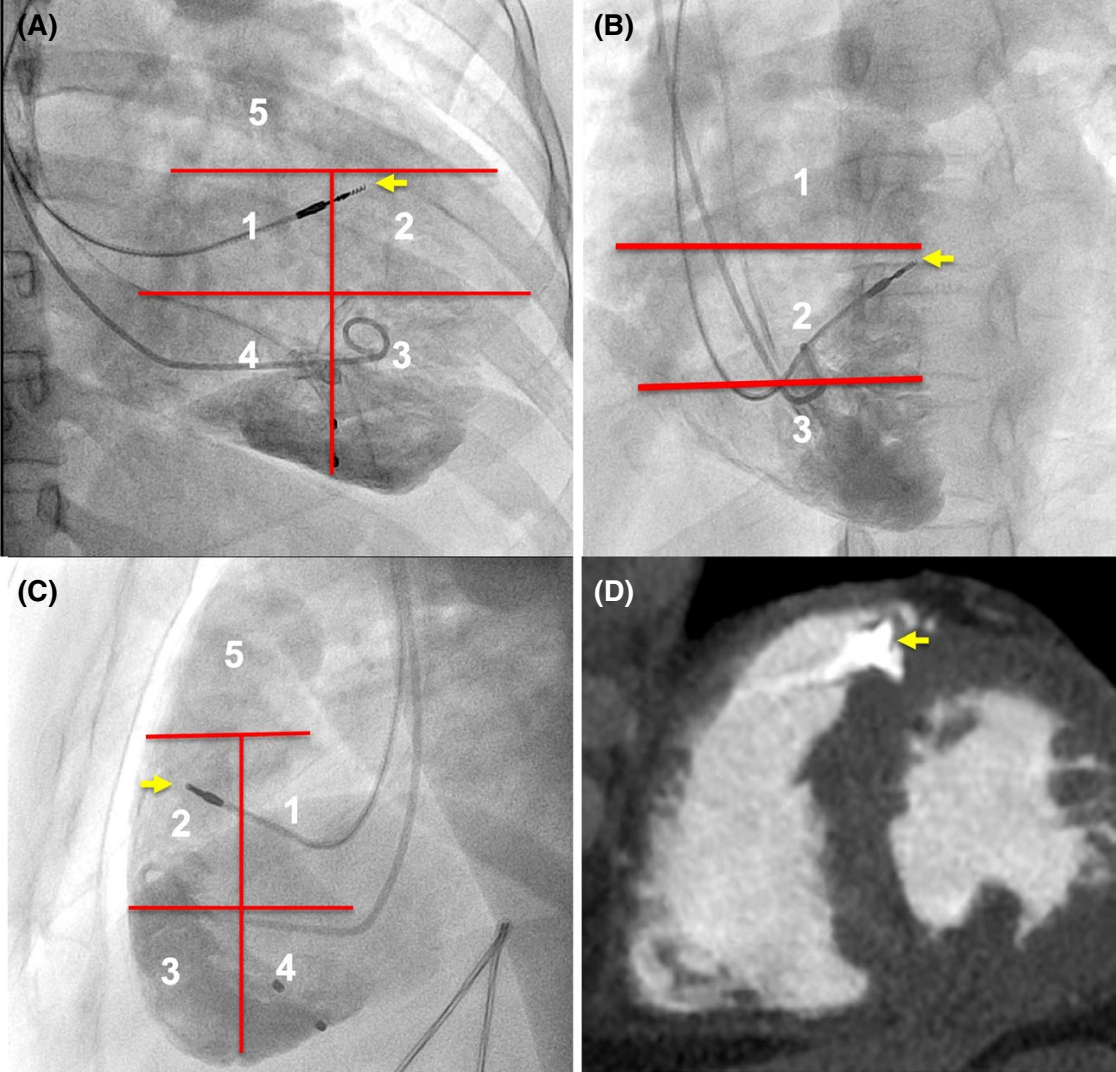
Right ventricular angiographic and CT scan of the anteroseptal lead position. RV angiography (A‐C) along with the described segments and the comparative CT scan (D) in diastole. Panel demonstrates (A) RAO 30° with 5 segments, (B) LAO 40° with 3 segments, (C) left lateral with 5 segments, and (D) CT scan. Note the tip of the ventricular lead (yellow arrow) in segment 2 in RAO 30° and LL views and the upper part of the middle segment in the LAO 40° view in panels (A‐C). The CT scan in the short axis view in (D) shows the lead tip in the anteroseptal region. LAO, left anterior oblique view; LL, left lateral 90° view; RAO, right anterior oblique view; RV, right ventricle
